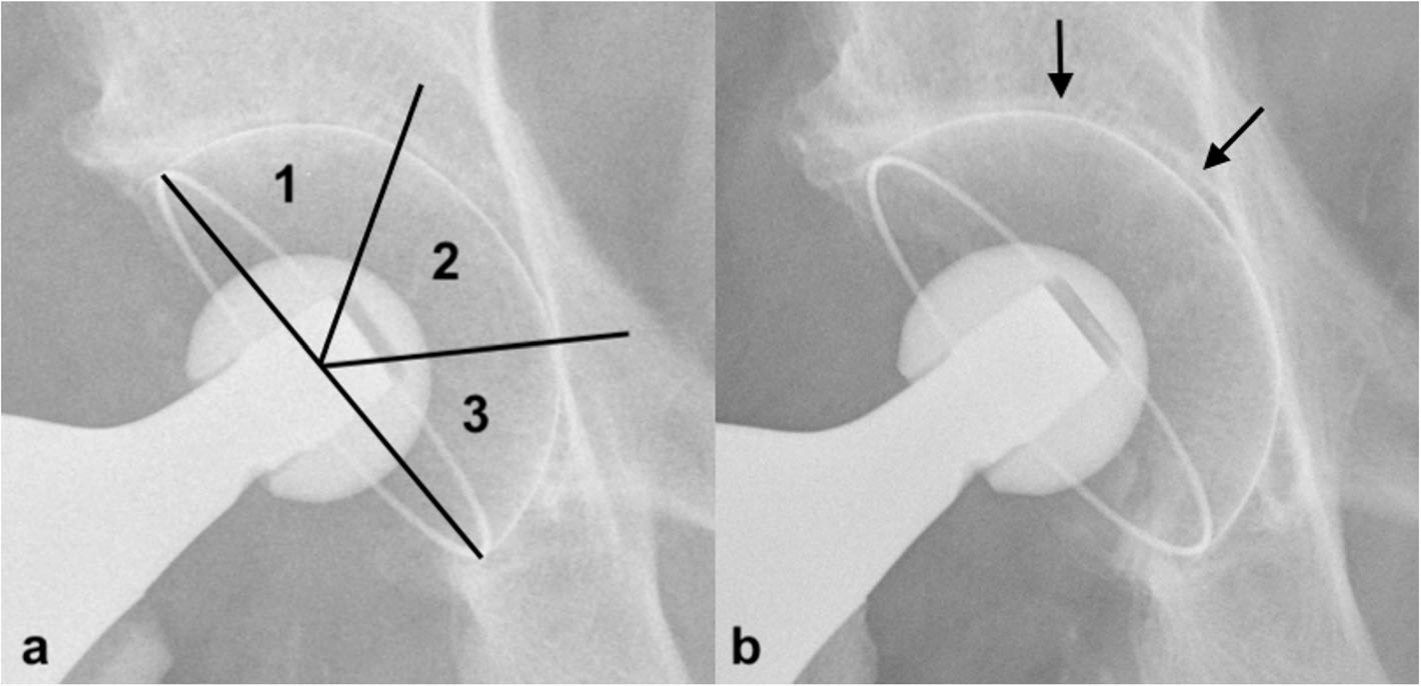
Radiographs of an RM Pressfit vitamys cup (Mathys Ltd. Bettlach, Switzerland) immediately after surgery (a) and at 5-year follow-up (b). The patient showed signs of a sclerotic line in Zones 1 and 2
